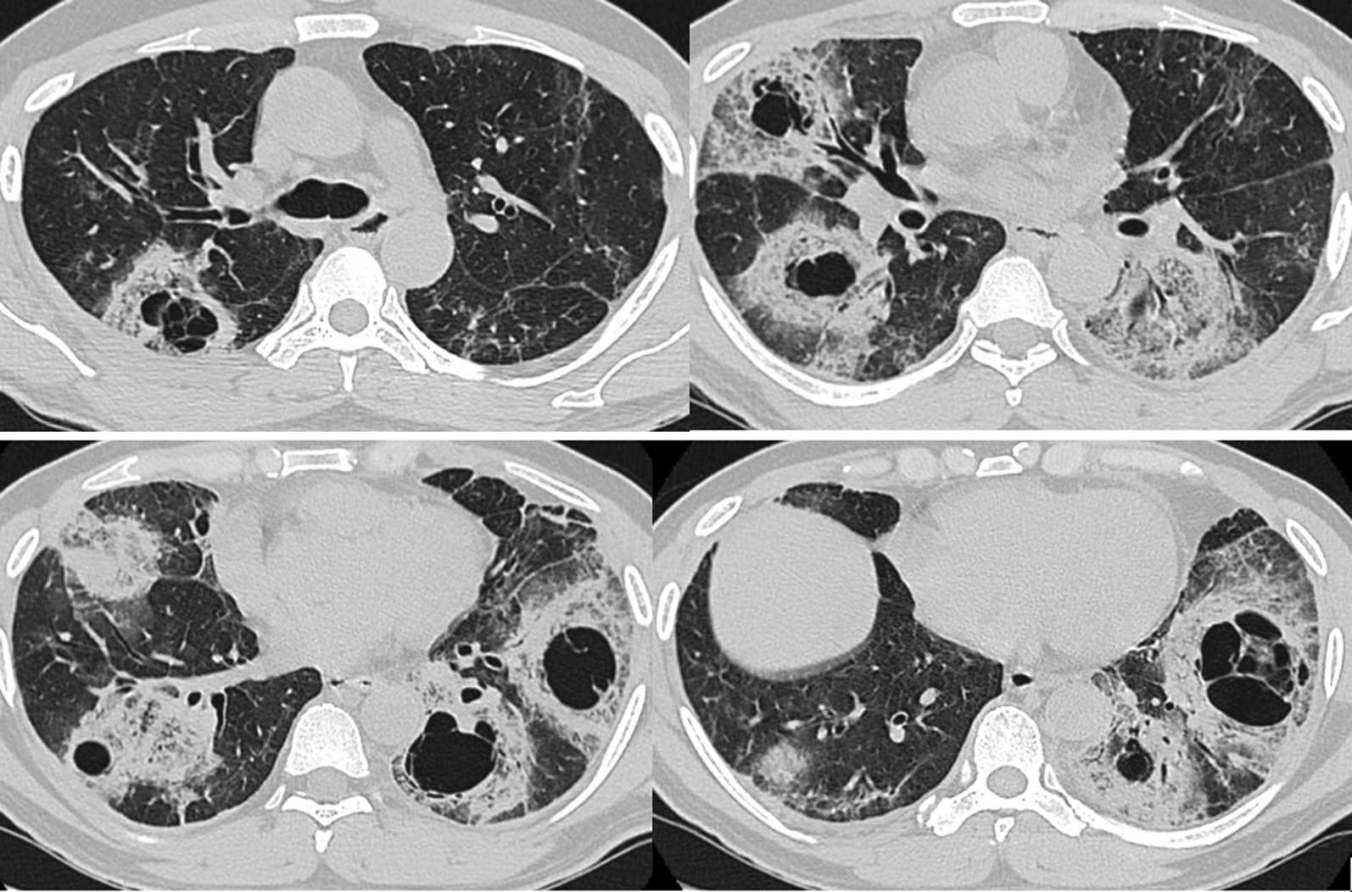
Axial HRCT scans showing imaging features of Mucormycosis in Case 3 of COVID-19. CT axial images shows multiple cavitary lesions with irregular nodular growth and internal septations, in both lungs, look like arising from pre-existing reverse halo sign
